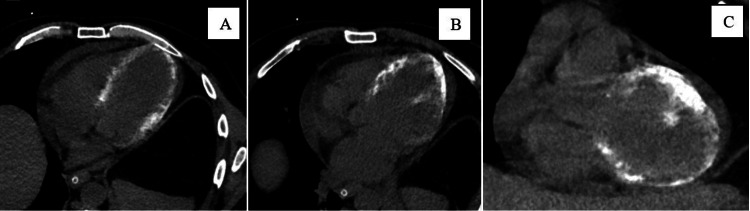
Non-contrast CT scan reveals the extensive myocardial calcifications in the LV wall. (A,B) Soft-tissue window, four-chamber view. (C) Soft-tissue window, short-axis view. CT: computed tomography; LV: left ventricular.
